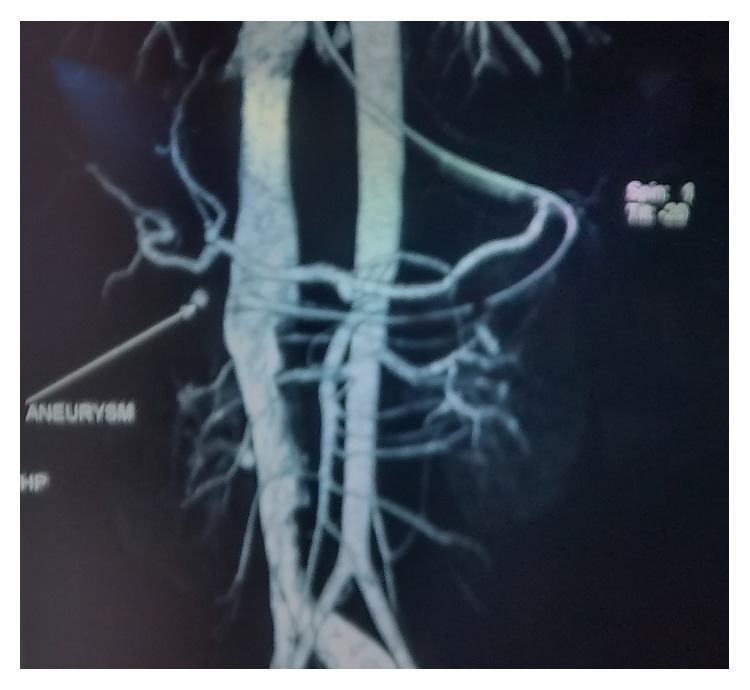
CT abdominal angiography showing the aneurysm of gastroduodenal artery.
